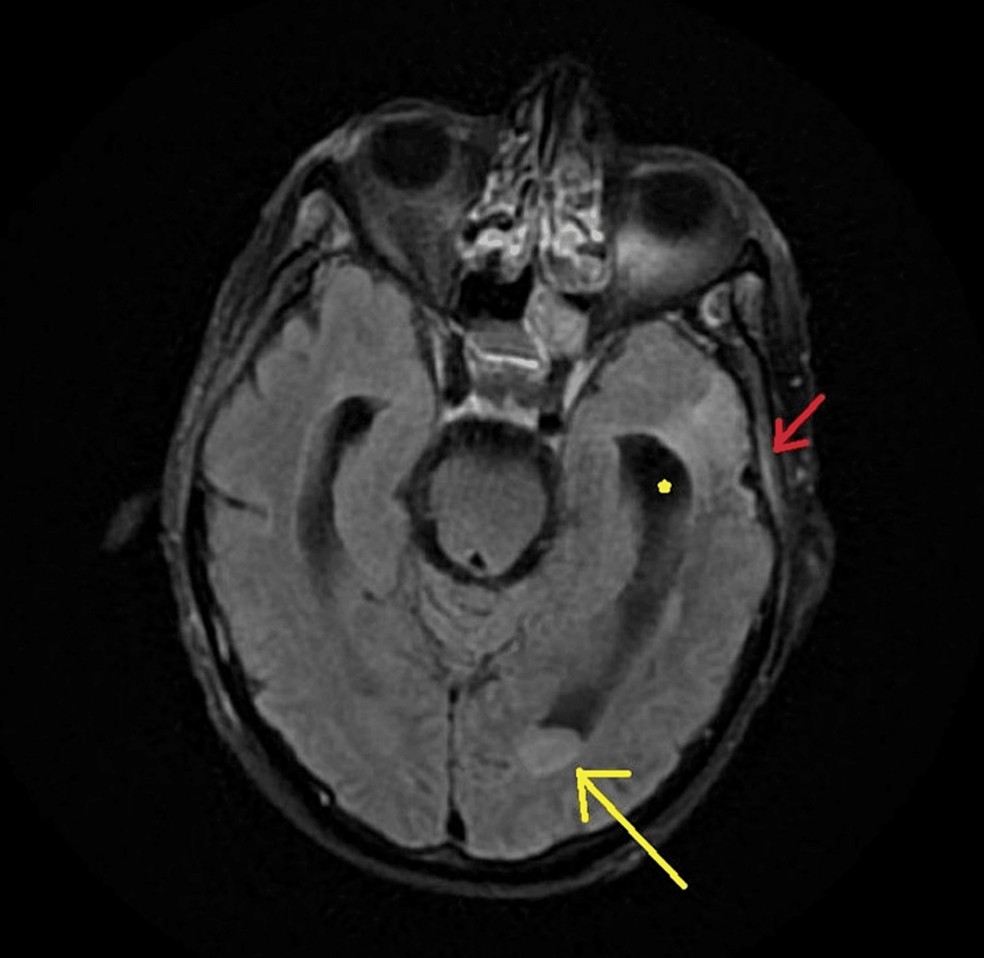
Magnetic resonance imaging fluid-attenuated inversion recovery (MRI FLAIR) showing debris in the posterior horn of the lateral ventricle with enhanced margins (yellow arrow), with ventriculomegaly (yellow star) and meningeal enhancement overlying left parietal lobe (red arrow) indicating meningitis.
